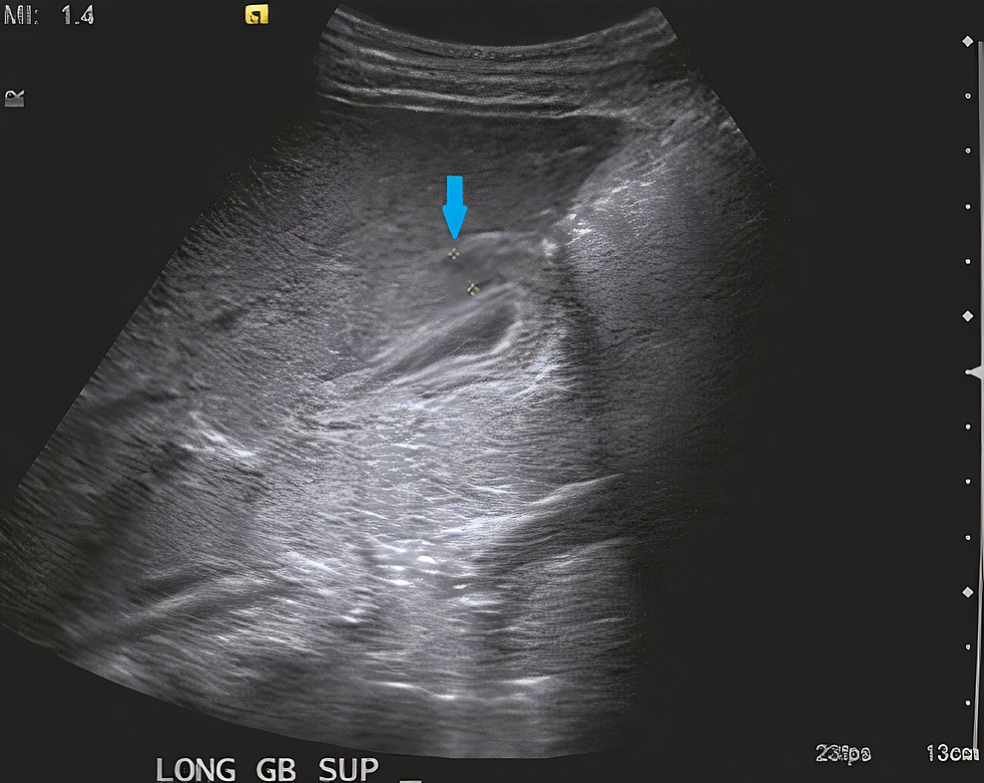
Abdominal ultrasound demonstrating gallbladder wall (blue arrow) of thickness 0.73 cm (yellow dashed crosses).
